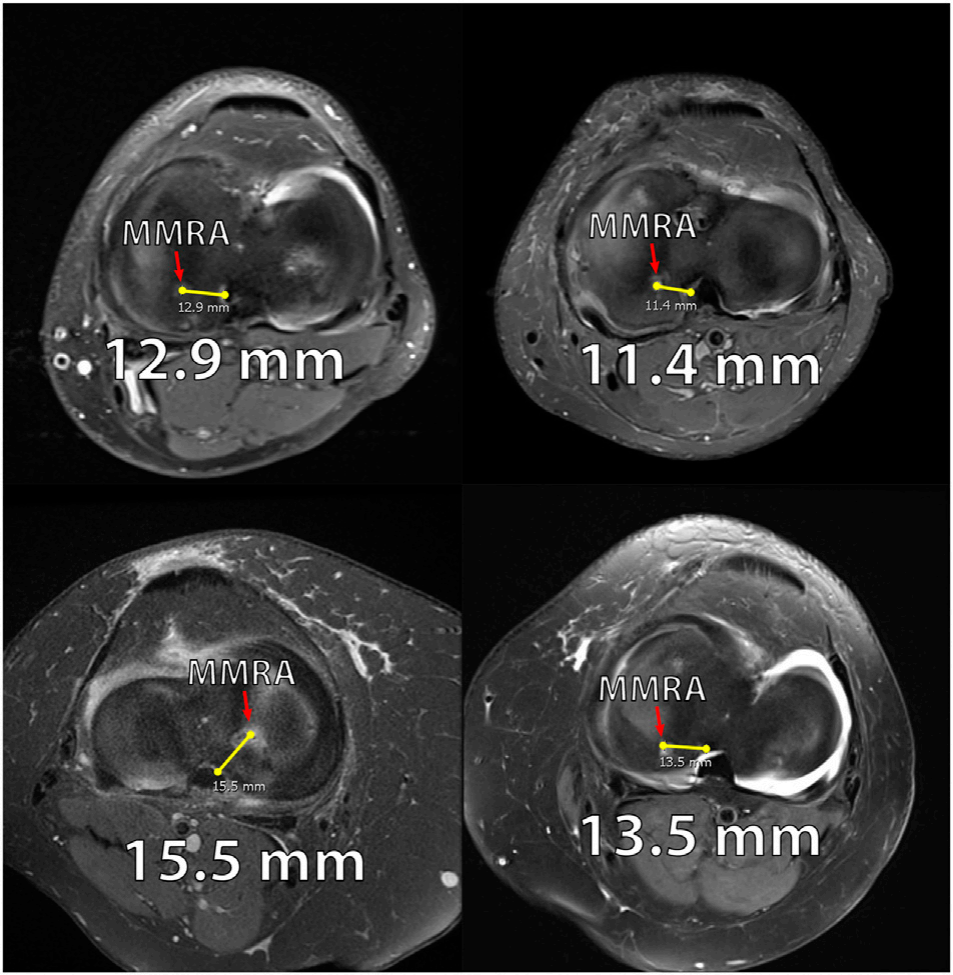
Axial MRIs of 4 patients from the nonanatomic meniscal root reconstruction cohort. (A): MRI of Nonanatomic Patient #1 displaying the medial meniscus root attachment 12.9 mm from anatomic attachment site (right knee). (B): MRI of Nonanatomic Patient #5 displaying the medial meniscus root attachment 11.4 mm from anatomic attachment site (right knee). (C): MRI of Nonanatomic Patient #4 displaying the medial meniscus root attachment 15.5 mm from anatomic attachment site (left knee). (D). MRI of Nonanatomic Patient #6 displaying the medial meniscus root attachment 13.5 mm from anatomic attachment site (right knee).
